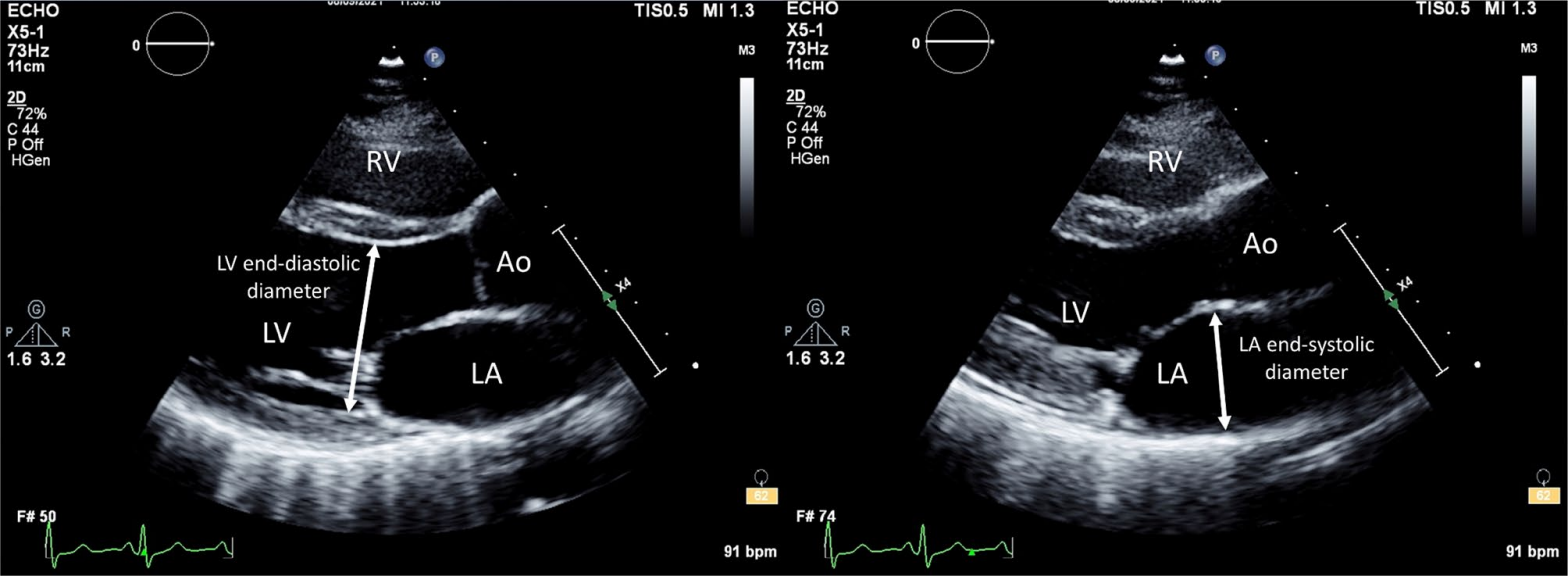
Measurement of atrioventricular area difference in transthoracic echocardiography. In routine echocardiography, atrioventricular area difference can be calculated as the difference between left ventricular end-diastolic short-axis cross-sectional area and left atrial end-systolic short-axis cross-sectional area. Short-axis cross-sectional area is calculated by circular approximation using left ventricular end-diastolic diameter and left atrial end-systolic diameter measured in a parasternal view. The atrial short-axis and ventricular short-axis areas represent the surface areas which contribute to the generation of hydraulic forces which contribute to the diastolic longitudinal motion of the atrioventricular plane. Abbreviations: Ao = aorta; LA = left atrium; LV = left ventricle; RV = right ventricle.
